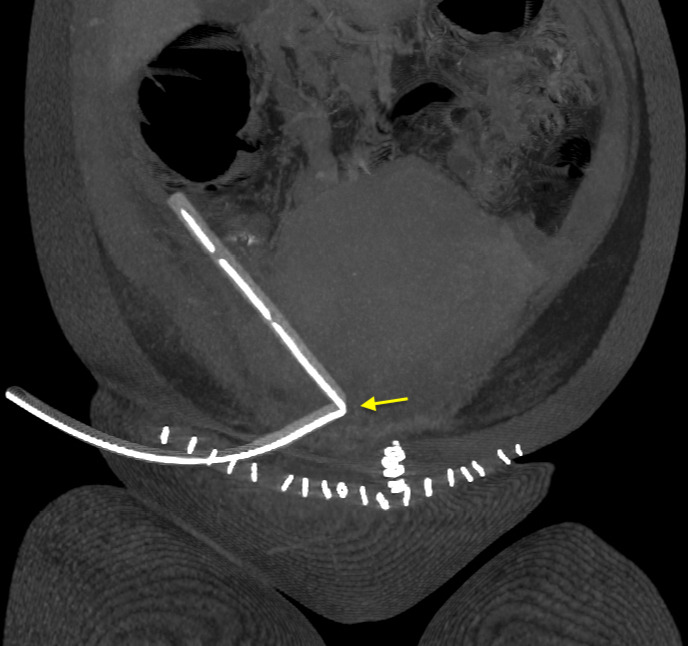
Coronal CT abdomen and pelvis showing Robinson drain on the right side of the pelvis positioned with the tip intraperitoneally in the right flank. A 90-degree angulation (arrow) in the surgical drain as it traverses through the rectus sheath.
